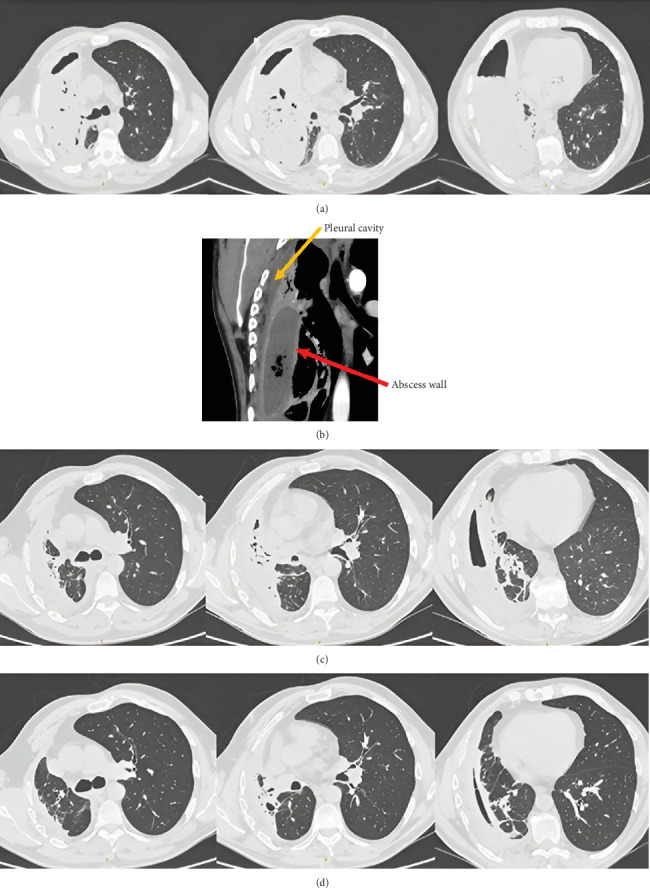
Follow-up CT scans performed on the (a) 1st of September 2022, (b) 6th of October 2022, (c) 25th of October 2022, and (d) 28th of December 2022—Case 2.
